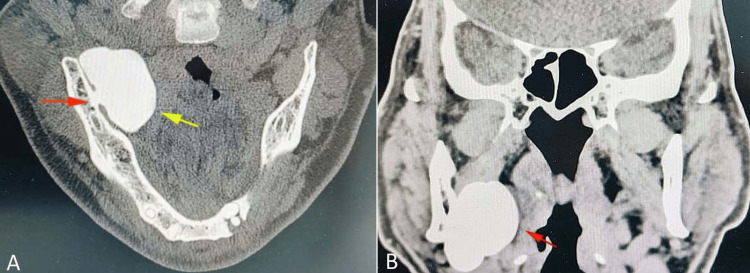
Cone-beam computed tomographic (CBCT) image of the mandible. (A) Axial view showing radiopaque lesion (yellow arrow) attached with stalk (red arrow) on the medial aspect of the right posterior mandible. (B) Coronal view showing radiopaque lesion (red arrow) on the medial surface of the right posterior mandible causing mild displacement of adjacent soft tissues but no invasion. Original CBCT image of the patient, used with patient's permission.
